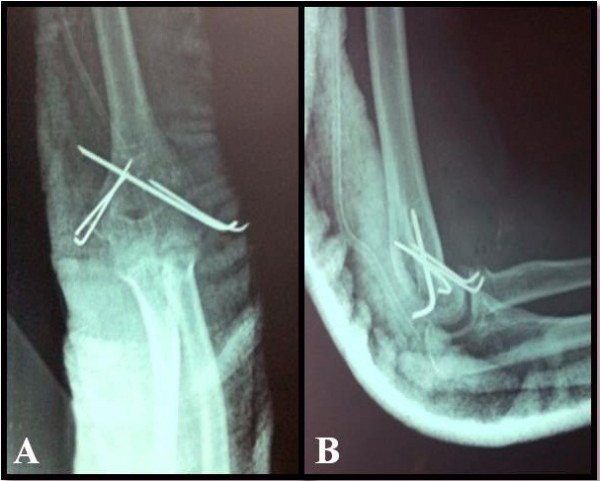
Postoperative anteroposterior and lateral X-ray views of the left elbow.
